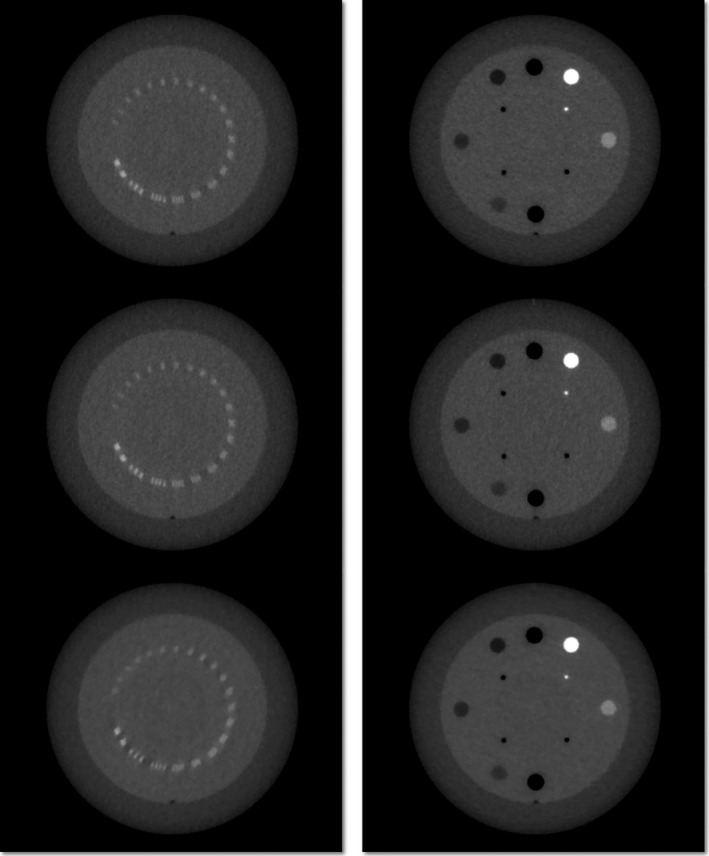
Computed tomography slices for normal pitch megavoltage computed tomography (2 mm slice thickness) using standard (row 1), general iterative (row 2), and soft‐tissue iterative (row 3) image reconstruction techniques. Spatial resolution worsens and noise decreases from standard to soft‐tissue image reconstruction, while contrast (right column) appears comparable.
